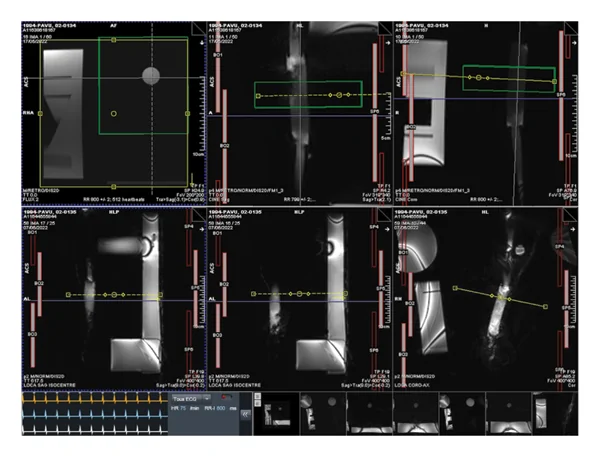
MRI processing interface of PC and SSFP sequences. The image shows the plane of interest in the axial, coronal, and sagittal axes of the CMBPT.
